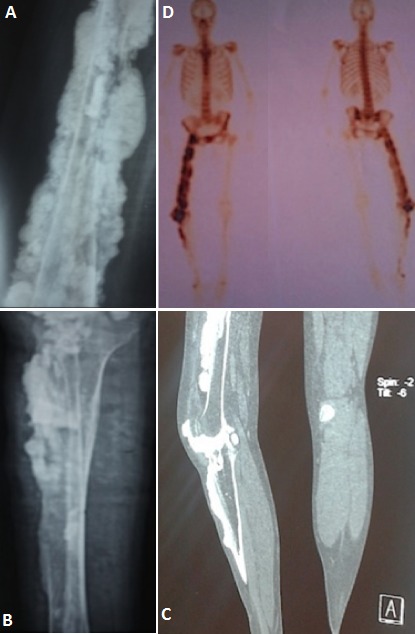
(A) radiographie du fémur droit, retrouve des opacités longilignes en «coulées de bougie» s’étendant le long de la corticale fémorale droite avec de multiples foyers d'ostéoscléroses nodulaires et ossification des tissus mou para articulaires et osseux; (B) radiographie standard de la jambe droite, en faveur d'opacitéslongilignes tibio-peronière associées à des foyers d'ostéoscléroses nodulaires; (C) scanner des membres inférieurs, montre des hypercondensations linéaires en «coulées de bougie», étendues de la corticale du fémur droit, bien limitées, parallèle au grand axe de l'os, avec comblement par endroit de la médullaire; (D) cintigraphie osseuse, hyperfixation osseuse le long du fémur droit et de l'extrémité supérieure de la jambe du même côté
